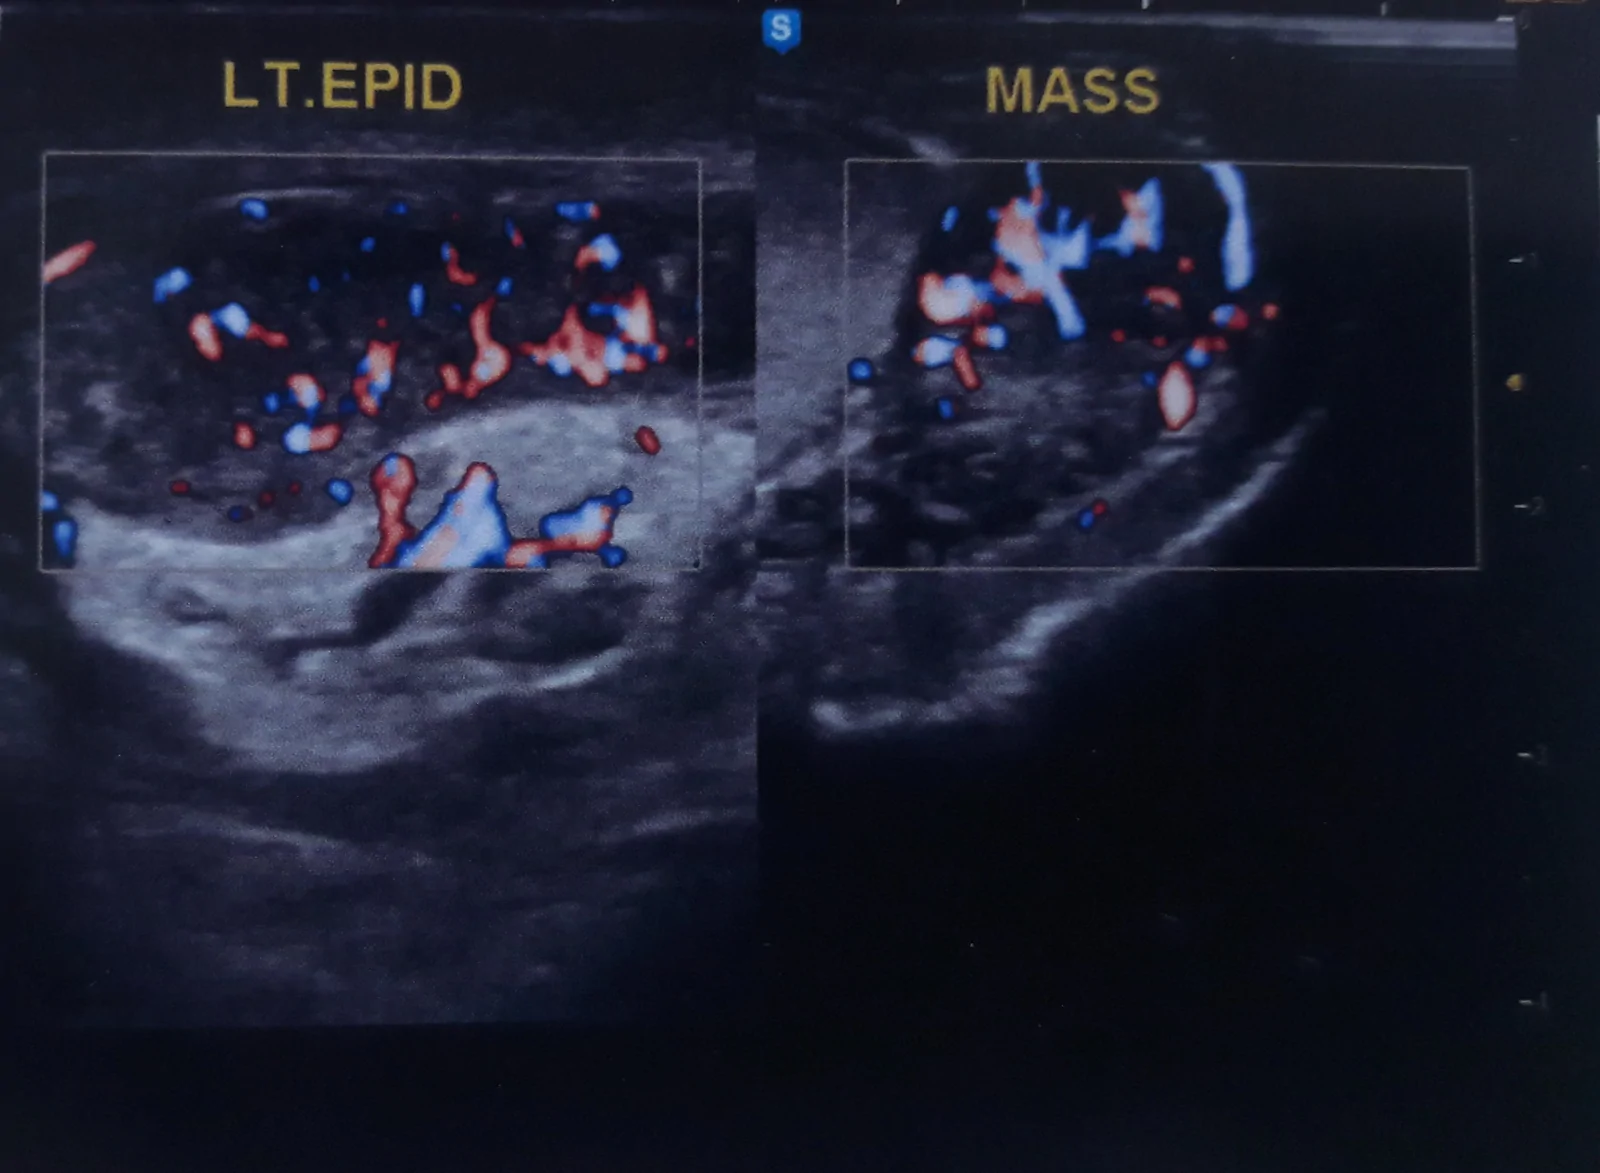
Scrotal Doppler showing increased vascularity in left epididymis of case 1. LT = left; EPID = epididymis.
